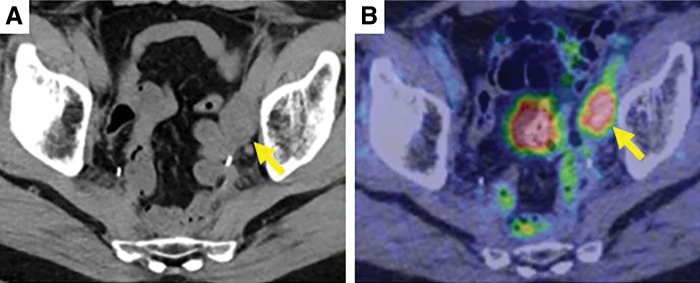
Plain CT and PET-CT images taken 7 years after the initial surgery. CT scan shows a 25-mm enlarged lymph node (arrow) (A), and PET-CT shows accumulation of SUVmax 8.06 (arrow) (B).
